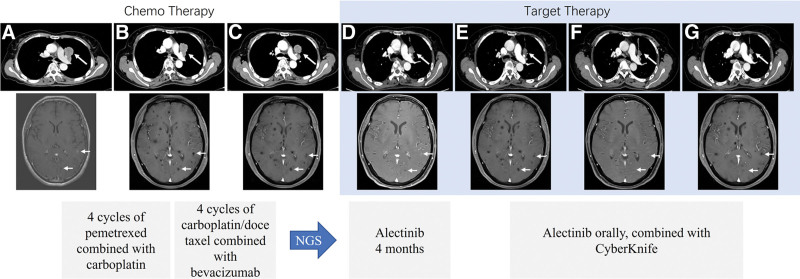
Dynamic chest CT scan (up), brain MRI imaging (down) and treatment timeline of patients during treatment. Chest CT and brain MRI at initial diagnosis (A). After 4 cycles of pemetrexed combined with carboplatin treatment, the size of left upper lung lesions decreased, but the number of brain lesions increased (B). After 4 cycles of carboplatin/docetaxel combined with bevacizumab treatment, the size of the left upper lung lesion was stable, but the brain lesion continued to deteriorate (C). Four months target therapy of treatment with Alectinib, the size of the left upper lung lesions was reduced, the brain lesions was significantly reduced in number and size (D). After 8 months of treatment with Alectinib, the left upper lung lesions remained unchanged. However, the number and size of brain lesions increased again (E). Take Alectinib orally combined with CyberKnife for 7 months, the left upper lung lesion was continually shrunk, the number and size of brain lesions were reduced (F). After 29 months of treatment with Alectinib combined with CyberKnife, the left upper lung lesions stayed stable, number of brain lesions increased slightly, but no local enhancement was found on enhanced MRI (G). CT = computed tomography, MRI = magnetic resonance imaging.
